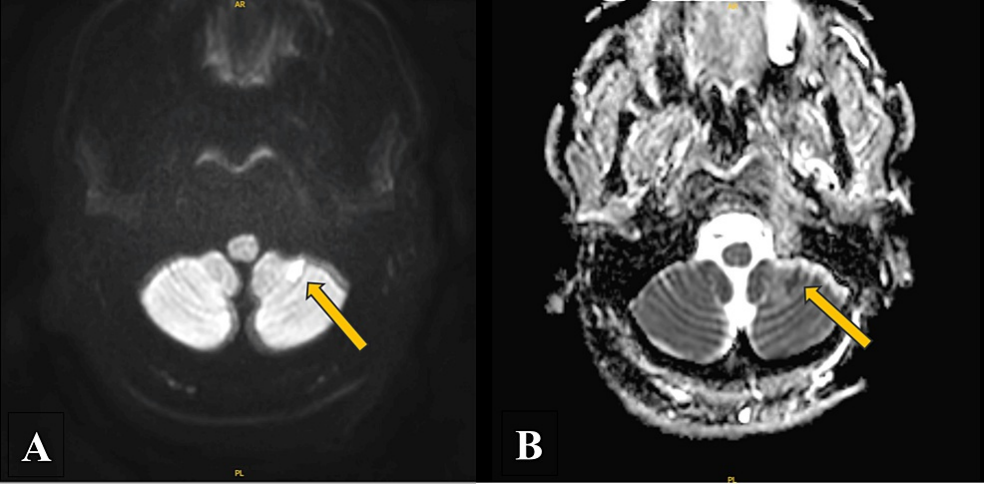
DWI MRI series DWI MRI series revealing a hypointense foci consistent with a recent small left cerebellar infarction. DWI: diffusion-weighted imaging
